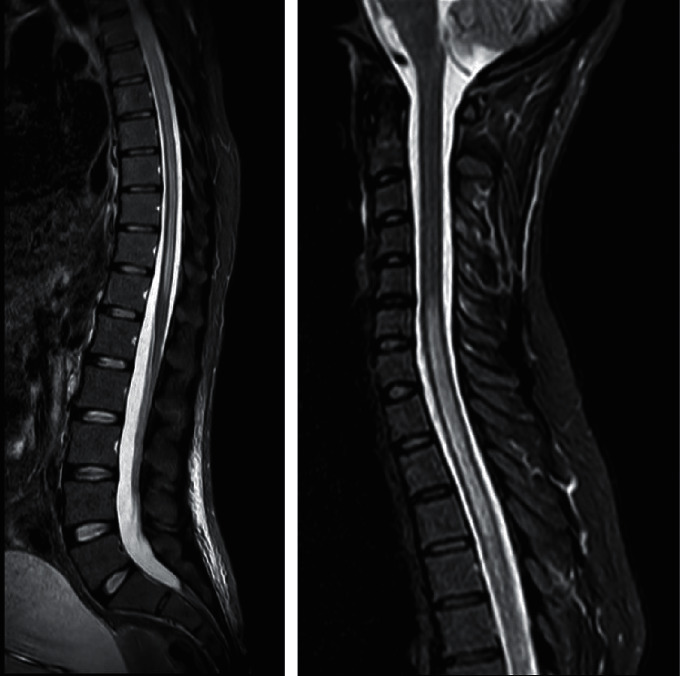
STIR view in magnetic resonance imaging of the patient's spinal cord indicates longitudinally extensive transverse myelitis.
